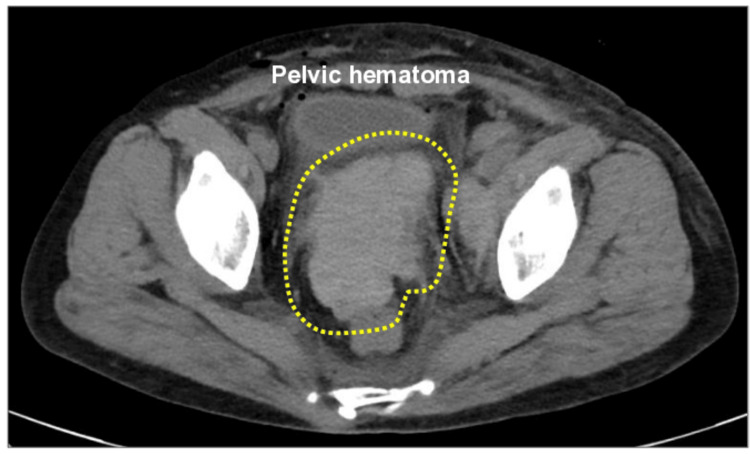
Postoperative pelvic hematoma without active bleeding. Contrast-enhanced computed tomography on postoperative day 3 showing a 7-cm pelvic hematoma (dotted outline) extending from the vaginal cuff toward the right uterine artery region, without evidence of contrast extravasation at this stage.
